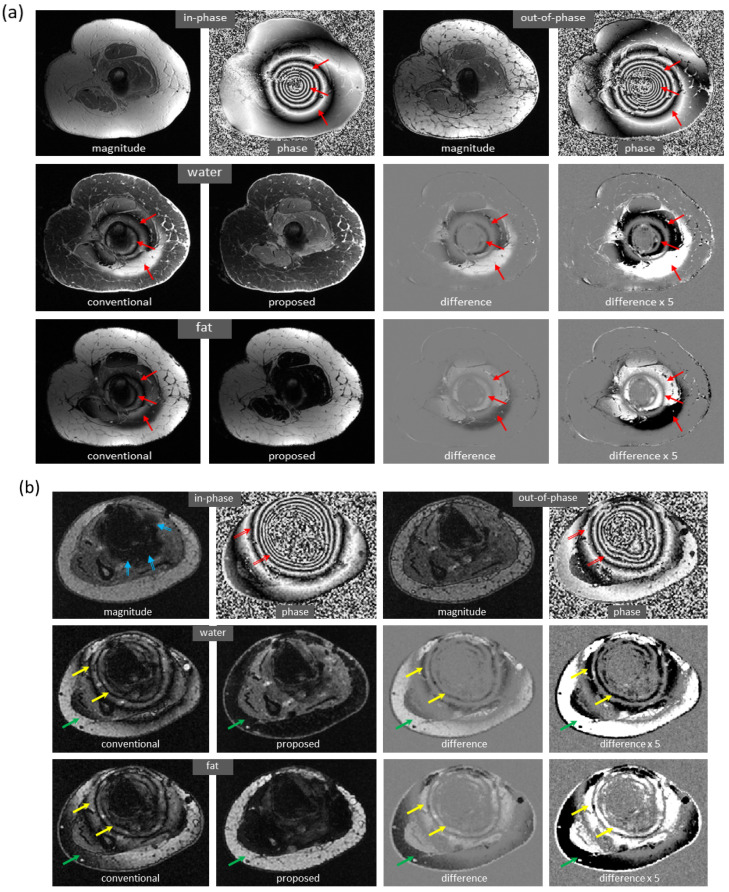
Marked reduction of metal-induced artifacts, where the training set lacked any examples with metallic implants. (a) In this knee case acquired on a PET-MRI scanner, severe off-resonance artifacts occurred in the reference images since the local magnetic field near metal changed dramatically (as can be seen from the input phase images). In the predicted images, metal-induced artifacts were largely corrected. (b) In another ankle case acquired on a 1.5T MRI scanner, signal loss (blue arrow) and phase corruption (red arrow) were observed in the input images, resulting in artifacts in the reference images (yellow arrow). In addition, severe water/fat swaps (green arrow) occurred in the peripheral region of this off-isocenter slice. Both metal artifacts and water/fat swaps were mitigated in the predicted images, even when the model was trained with only knee images acquired on 3T scanners.
